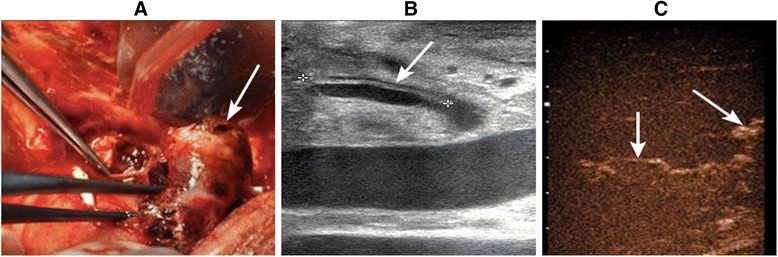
a A blood clot that was 4 × 3 cm in size was found in the hilus hepatis during urgent exploratory laparotomy (*arrow*). b Hepatic artery dissection was identified by gray-scale ultrasound. c Intraoperative CEUS revealed the opening of collateral circulation
